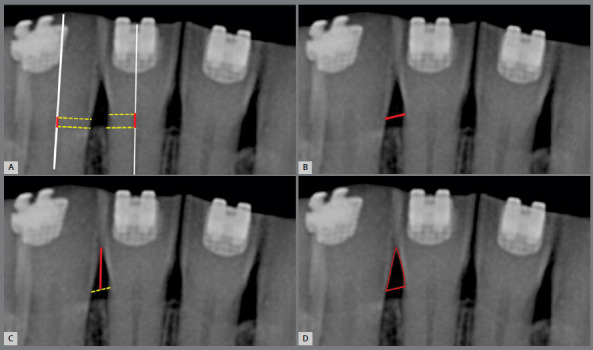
Determination of interproximal radiographic measurements of: height of interproximal bone (A), width of interproximal space (B), height of interproximal space (C) and area of interproximal space (D), represented by red lines.
